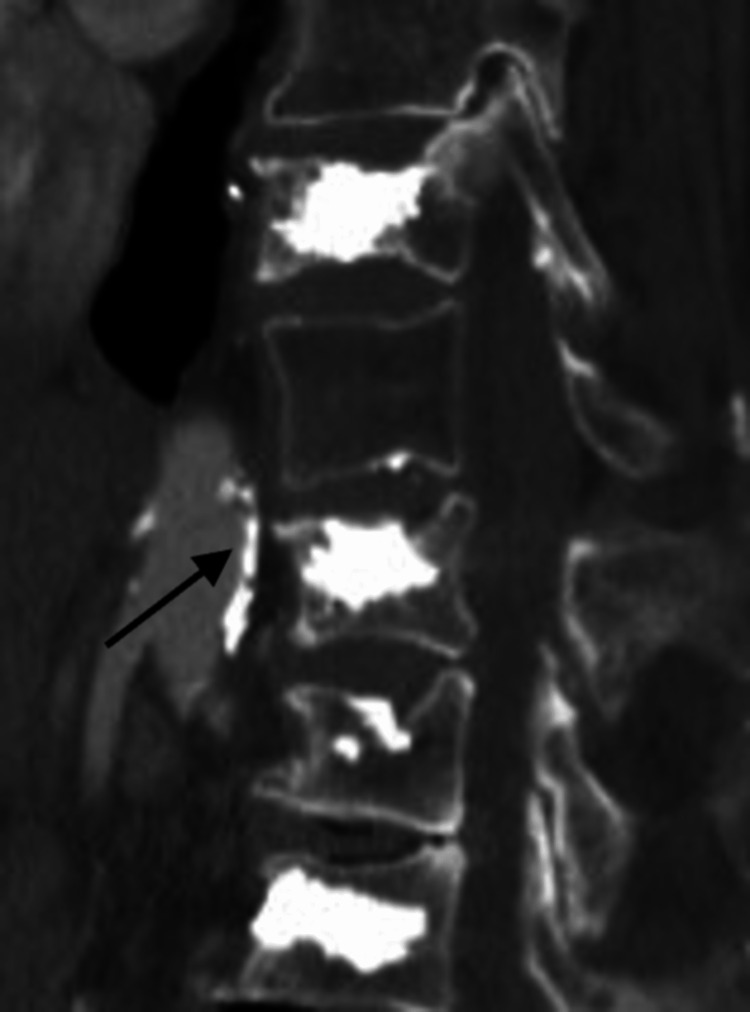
Computed tomography Hyper-dense material in the venous system adjacent to the L2 vertebroplasty location is denoted by the black arrow.
